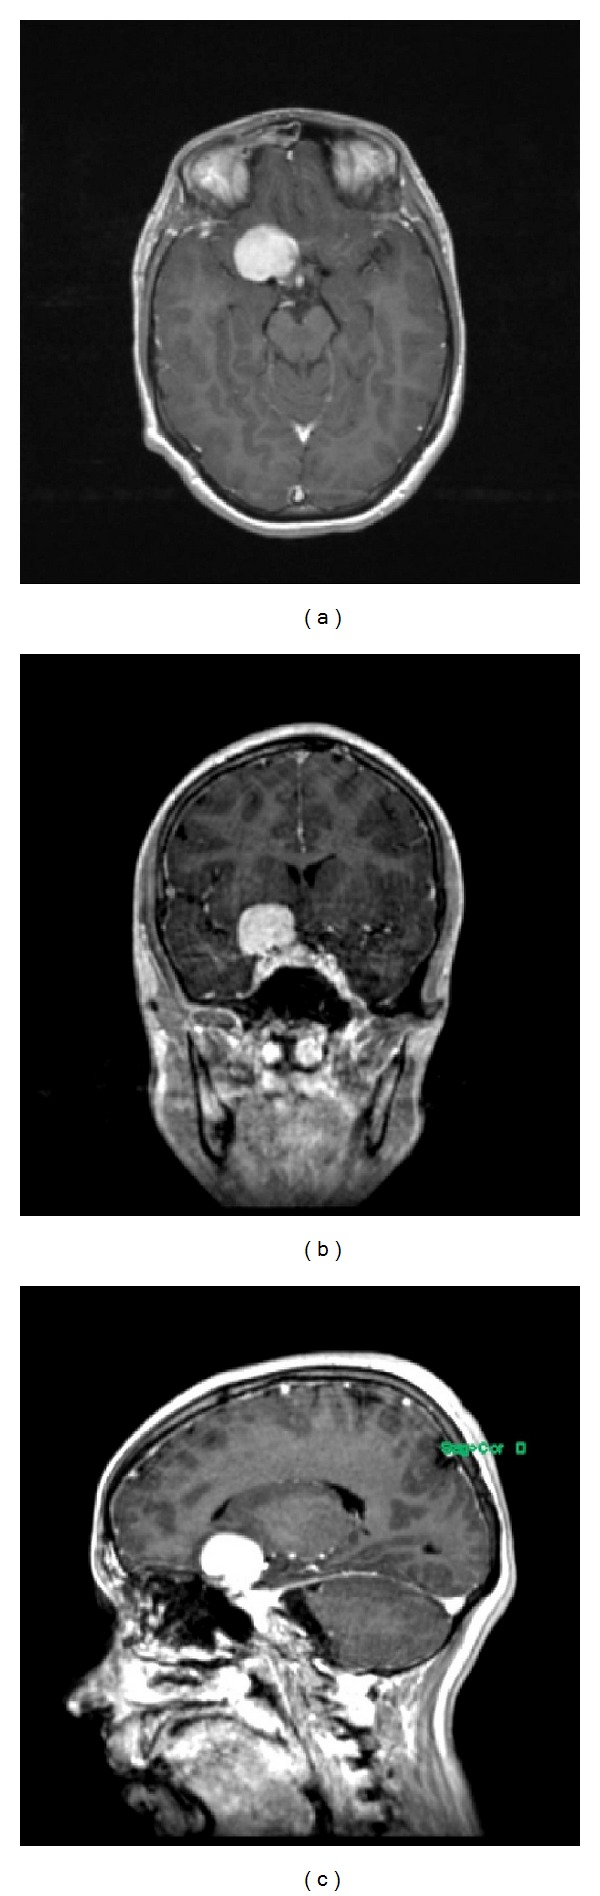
(a) Axial, (b) coronal, and (c) sagittal T1-weighted MRI showing a homogenously enhancing lesion, measuring 2.9 × 3.0 × 2.0 cm in the right clinoidal region. There is probable extension into the right optic canal, sphenoid sinus, right temporal fossa, and possibly right cavernous sinus, suggestive of a right clinoidal meningioma.
